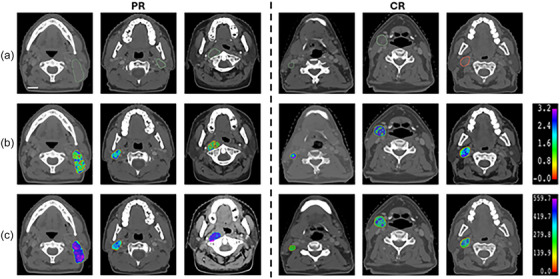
Representative CT radiomics features of three CR patients (On the left) and three PR patients (On the right). (a) Presents the CT image with ROIs. (b) GLDM‐ dependence entropy ([0–3.5] range). (c) GLCM cluster shade ([0–600] range). Scale bar is 5 mm.
